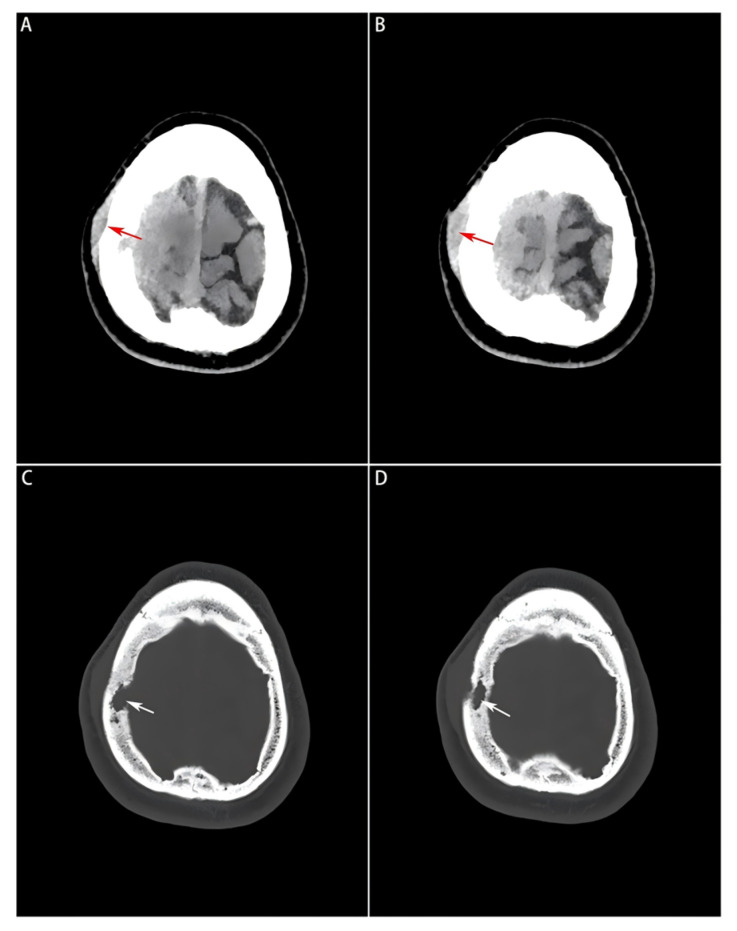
Preoperative head CT images of the soft tissue window and bone window. (A,B): A slightly high-density mass visualized on the soft tissue window, where the subcutaneous mass formed by the tumor lesion invading outside the bone window is indicated by a red arrow; (C,D): Bone erosion and defect visualized on the bone window image, and localized by a white arrow.
